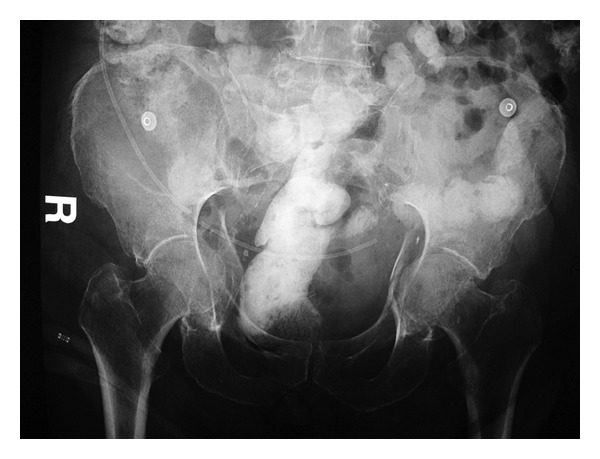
AP Pelvis X-ray showing displaced fractures of both acetabuli with intrapelvic protrusion of both femoral heads.
